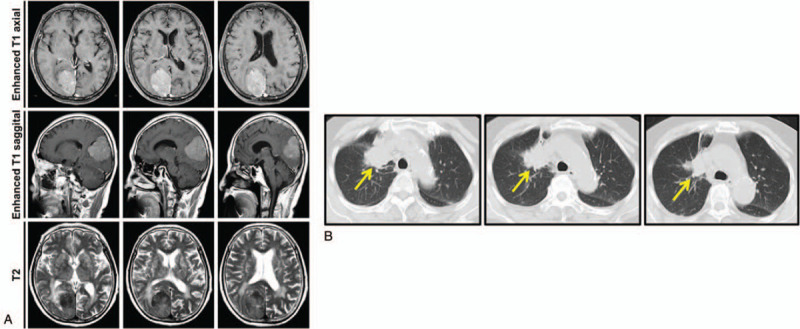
Imaging examinations of the brain and lungs before surgery. A, Preoperative contrast-enhanced MRI examination of the brain reveals a well-circumscribed mass (4.5 × 3.6 × 4.7 cm) in the right occipital parafalcine region. B, CT scan of the chest shows a 3.6 × 3.3 cm mass in the right upper lobe.
